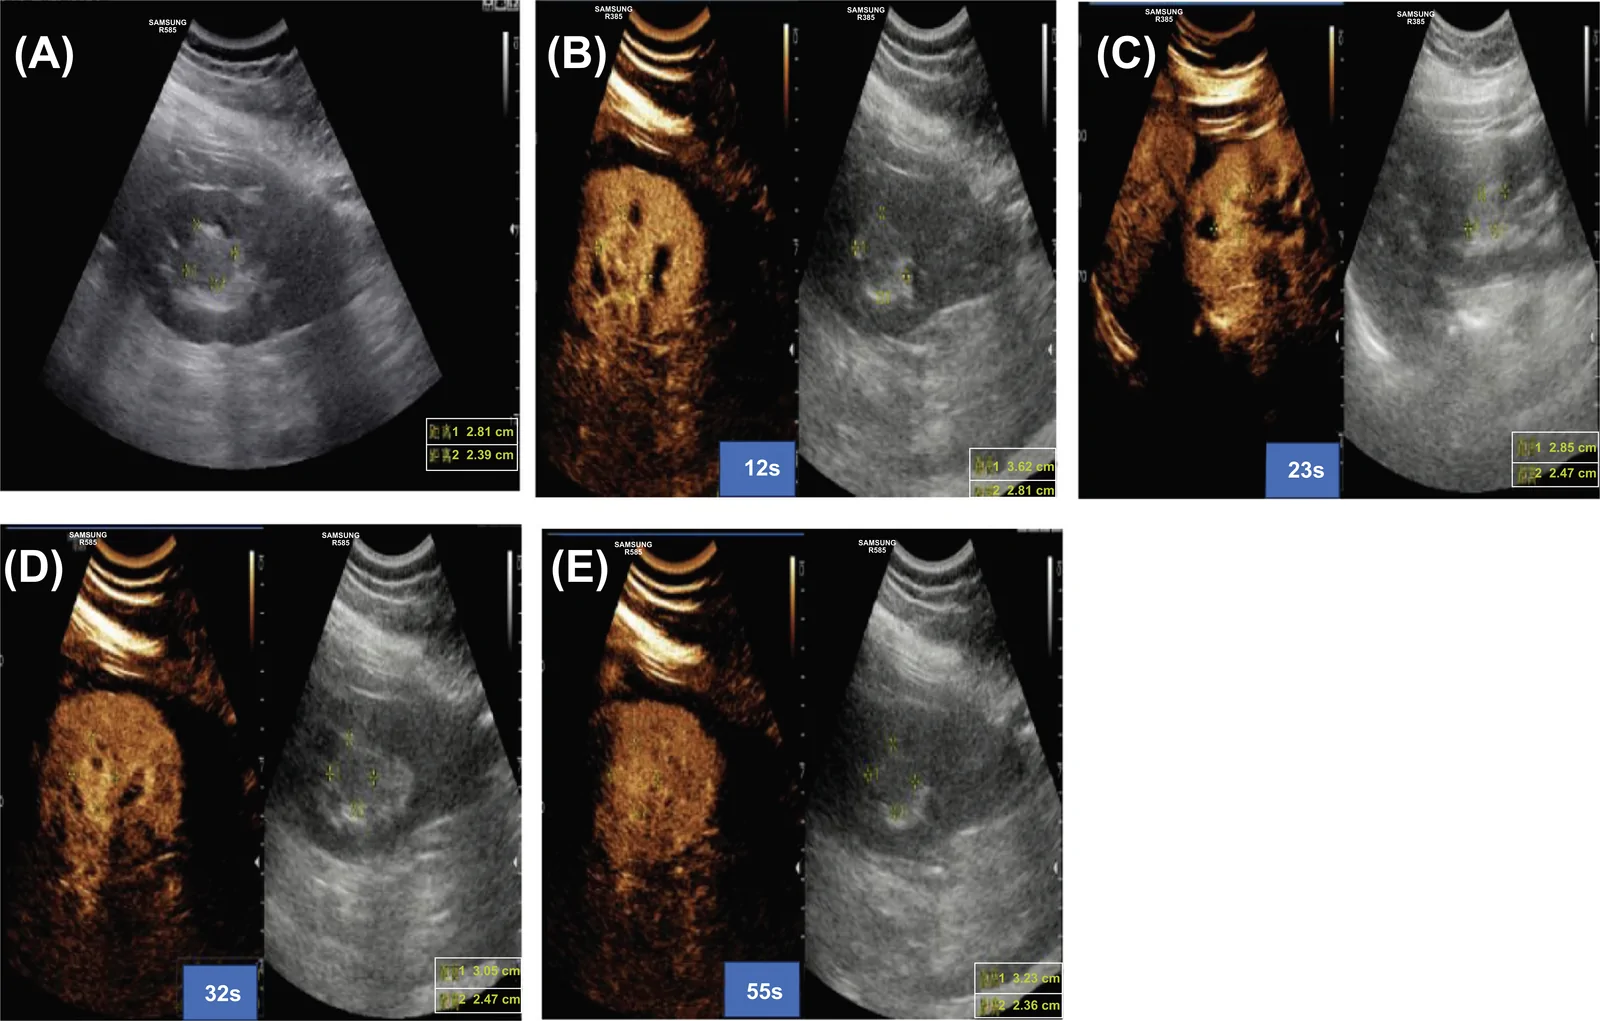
Patient Shen, a 50-year-old female. A: Two-dimensional ultrasound shows that the lesion is located within the left renal sinus, presenting as hypoechoic, with a size of approximately 3 × 2.7 cm, oval-shaped, and with relatively clear margins. B: At 12 seconds after bolus injection of SonoVue, the lesion shows peripheral nodular enhancement, the lesion in the left kidney shows nodular enhancement at the periphery during the cortical phase. C: At 23 seconds, the contrast agent enhances slowly from the periphery towards the center of the lesion. D: At 32 seconds, the contrast agent has completely filled the lesion, and at this time, the degree of enhancement of the lesion is slightly higher than that of the surrounding renal cortex. E: At 55 seconds, the contrast agent in the renal cortex begins to slowly fade, and at this time, the lesion in the left kidney appears slightly higher than the surrounding renal parenchyma.
